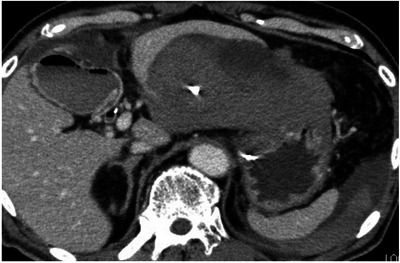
Bleeding after EUS‐HGS using a plastic stent. Computed tomography performed one day after EUS‐HGS showed a huge hematoma between the liver and stomach. This probably resulted from multiple punctures due to targeting of the small bile ducts. EUS‐HGS, endoscopic ultrasound‐guided hepaticogastrostomy.
